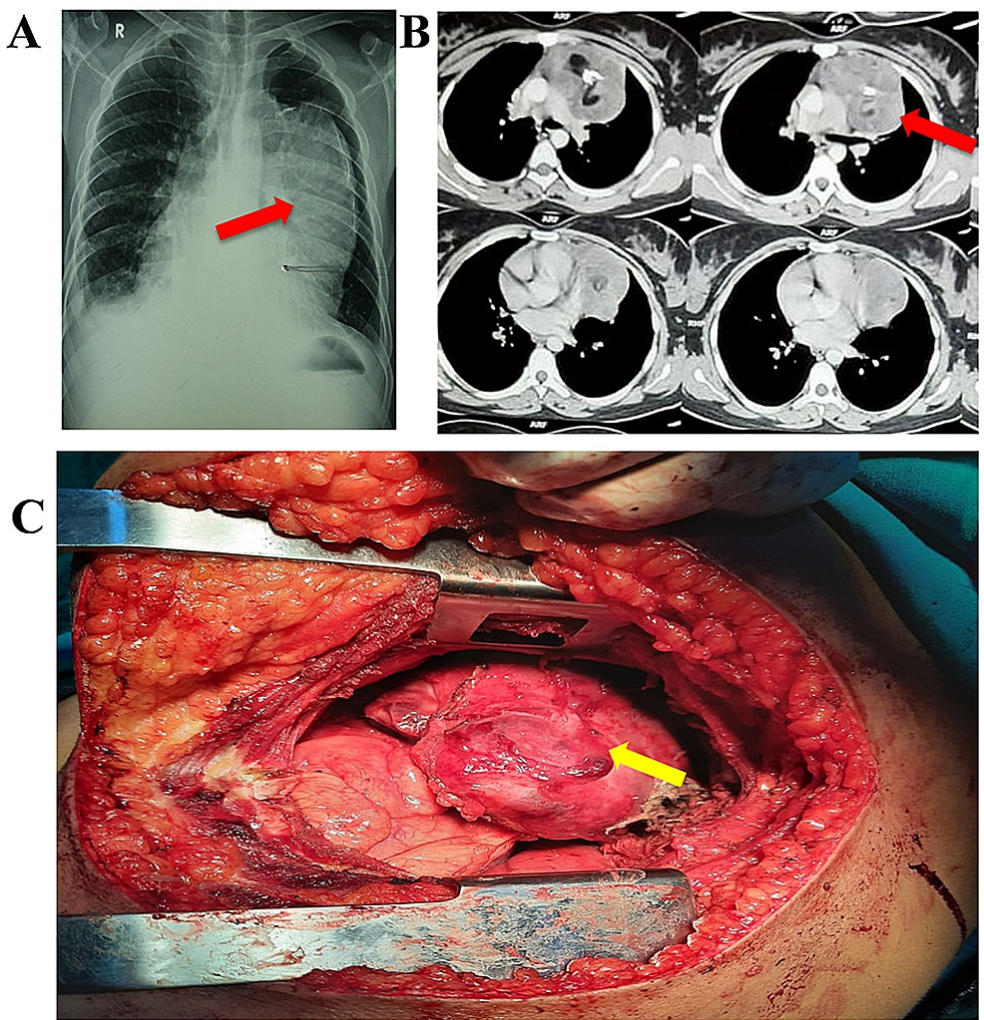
(A) Chest X-ray PA view; (B) contrast-enhanced CT of the chest; (C) Intraoperative picture. Red and yellow arrows represent benign teratoma in radiological and intraoperative figures, respectively. PA: posteroanterior; CT: computed tomography
